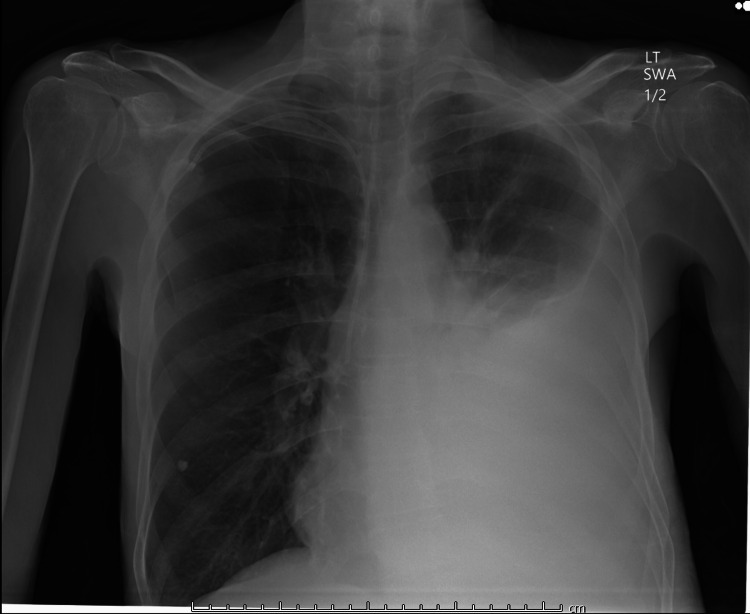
Patient's Chest X-ray two weeks ago showing left-sided pleural effusion
